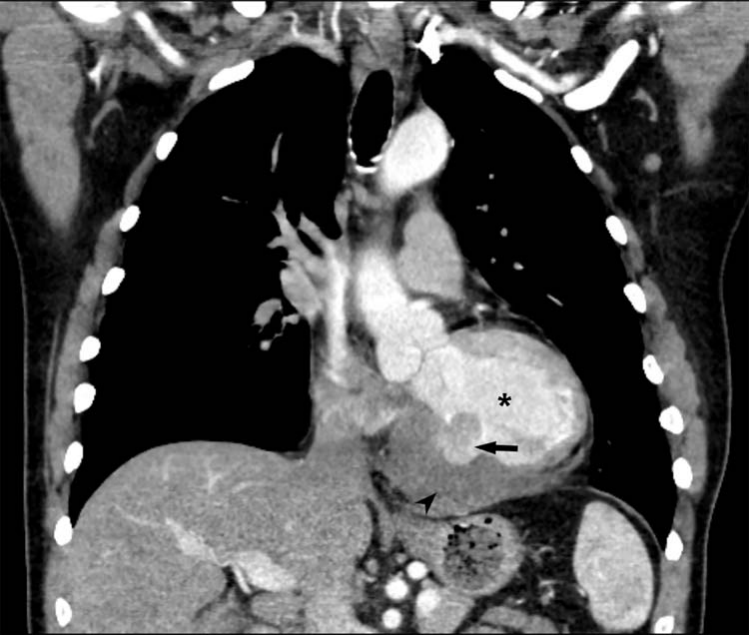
Coronal CT demonstrating left ventricular wall defect and intrapericardial thrombus; asterisk = left ventricular cavity, arrow = left ventricle free wall defect, arrowhead = thrombus.
